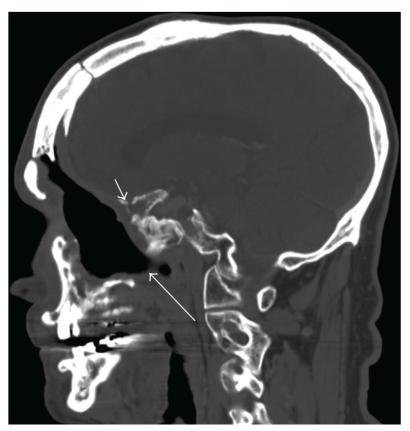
Radiation necrosis of the skull base with fistulous tract: Sagittal contrast-enhanced CT of the mandible bone (bone window) shows irregularity of the skull base (small arrow). In addition, there is a fistulous tract extending towards the oral cavity (large arrow).
